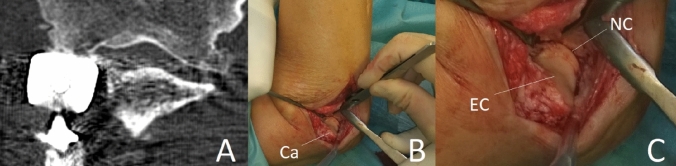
a CT-Scan of overlengthened radial head implant, overreaching the lateral edge of the coronoid—with erosion of the capitulum. b Intra-operative (Ca: Capitulum). c Magnification of the intra-operative findings. *EC* eroded cartilage, *NC* native cartilage of the trochlea
